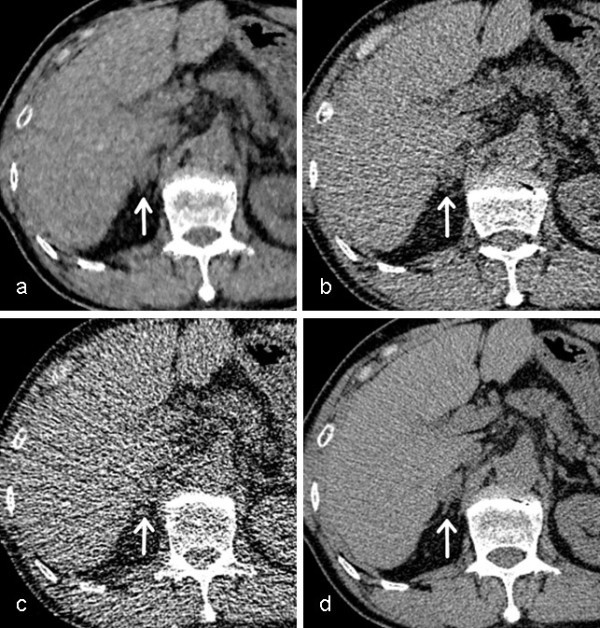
The adrenal nodule in each CT image. An adrenal nodule with 20 mm in longest diameter (white arrow) in axial unenhanced CT images of UL-MBIR (a), L-ASIR (b), UL-ASIR (c) and R-FBP (d) of a 65-year-old man weighing 61 kg. In UL-ASIR (c), the margin of this adrenal nodule is obscure due to the prominent image noise. The certainty level (UL-MBIR/L-ASIR/UL-ASIR) for this lesion by each reader was 4 (lesion definitely present)/3(lesion probably present)/3 by reader 1 and 3/3/0(no lesion) by reader 2, respectively.
